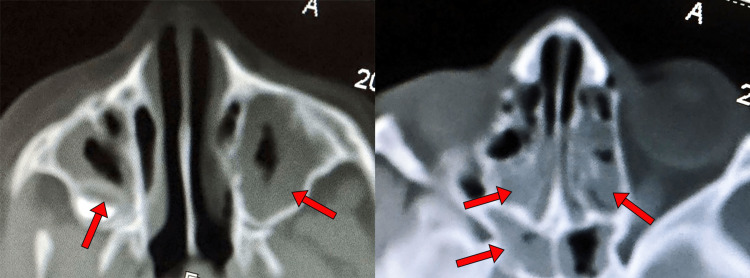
Ethmoidal and maxillary sinusitis by CT scan
